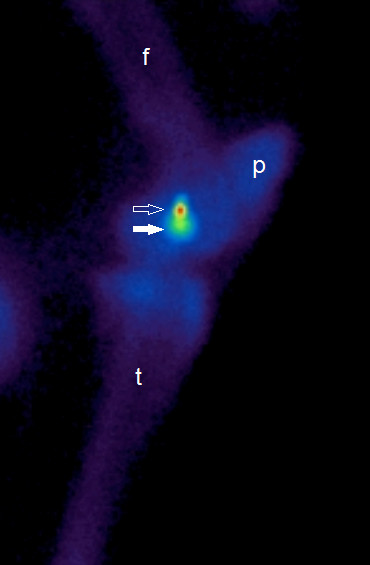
A lateral stifle scintigram showing vertical alignment of radioactive point sources. Due to increased distance to the gamma camera, the definition of medial point source (arrow) was poorer than the lateral point source (white arrow). In this image, the lateral point source is proximal to the medial one. Femur (f), patella (p) and tibia (t).
